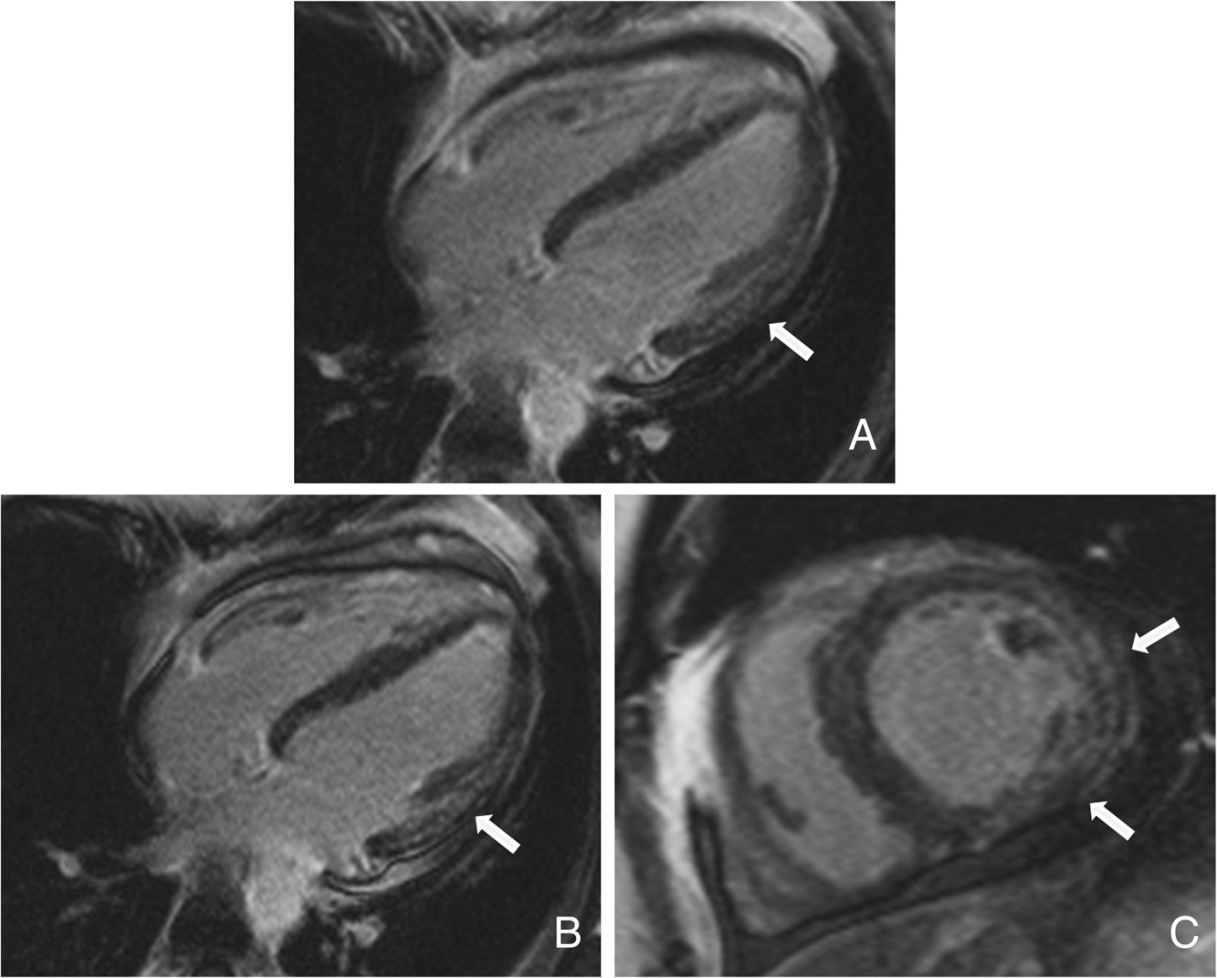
Cardiac magnetic resonance imaging (MRI), inversion recovery (IR) turbo fast low-angle shot (FLASH) sequence. (A) Beginning enhancement in lateral wall. (B-C) Intramural late-enhancement in lateral wall.
